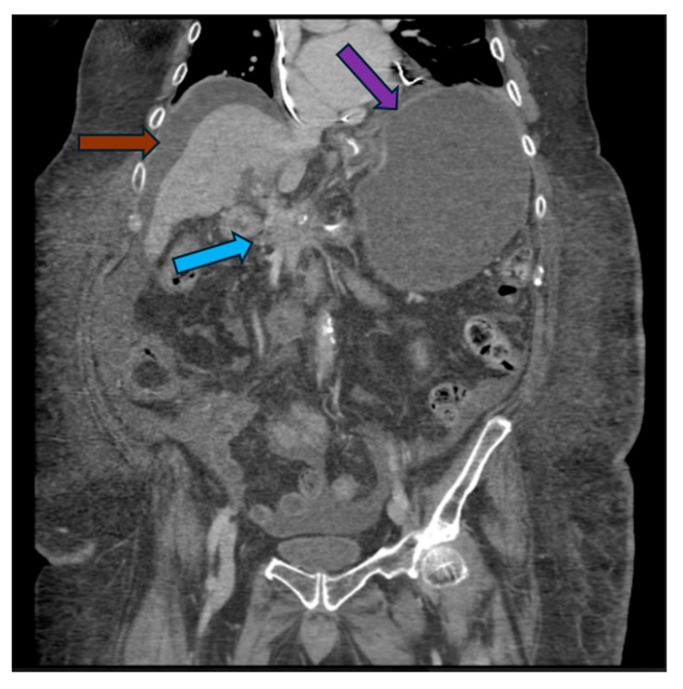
A coronal view of a computed tomography scan of the abdomen and pelvis showing an infiltrative pancreatic head mass (blue arrow) and a dilated, obstructed stomach (purple arrow). Ascitic fluid is demonstrated around the liver (brown arrow). Significant ascites usually precludes endoscopic ultrasound-guided gastroenterostomy.
